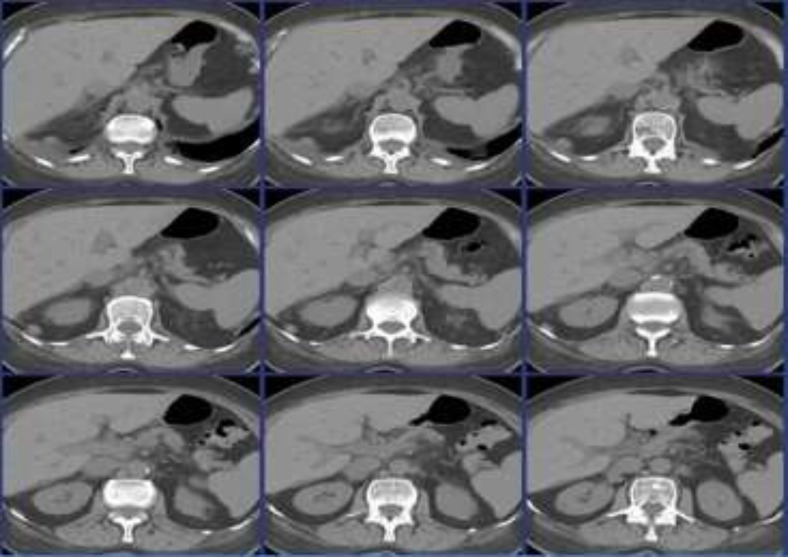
Atrophic morphology of the adrenal glands in contrast CT imaging
